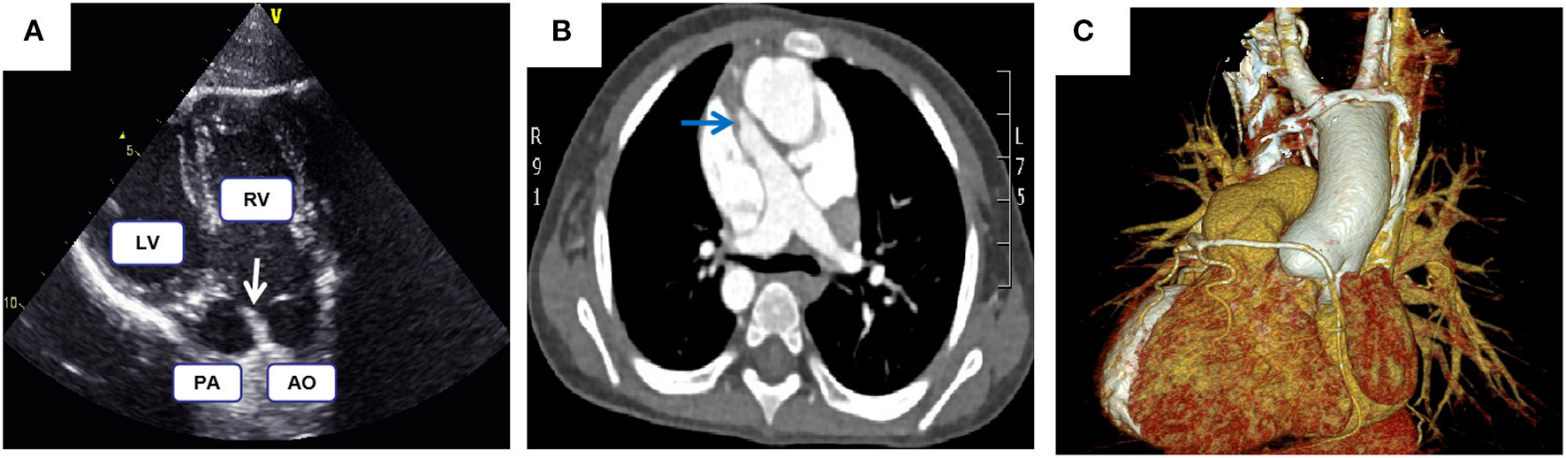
(A) Echocardiography demonstrates both the aorta and pulmonary artery originate from the right ventricle. (B,C) Computed tomography angiography shows malposed great vessels and anomalous right coronary artery looping around the pulmonary artery; Arrows highlight the pulmonary stenosis.
